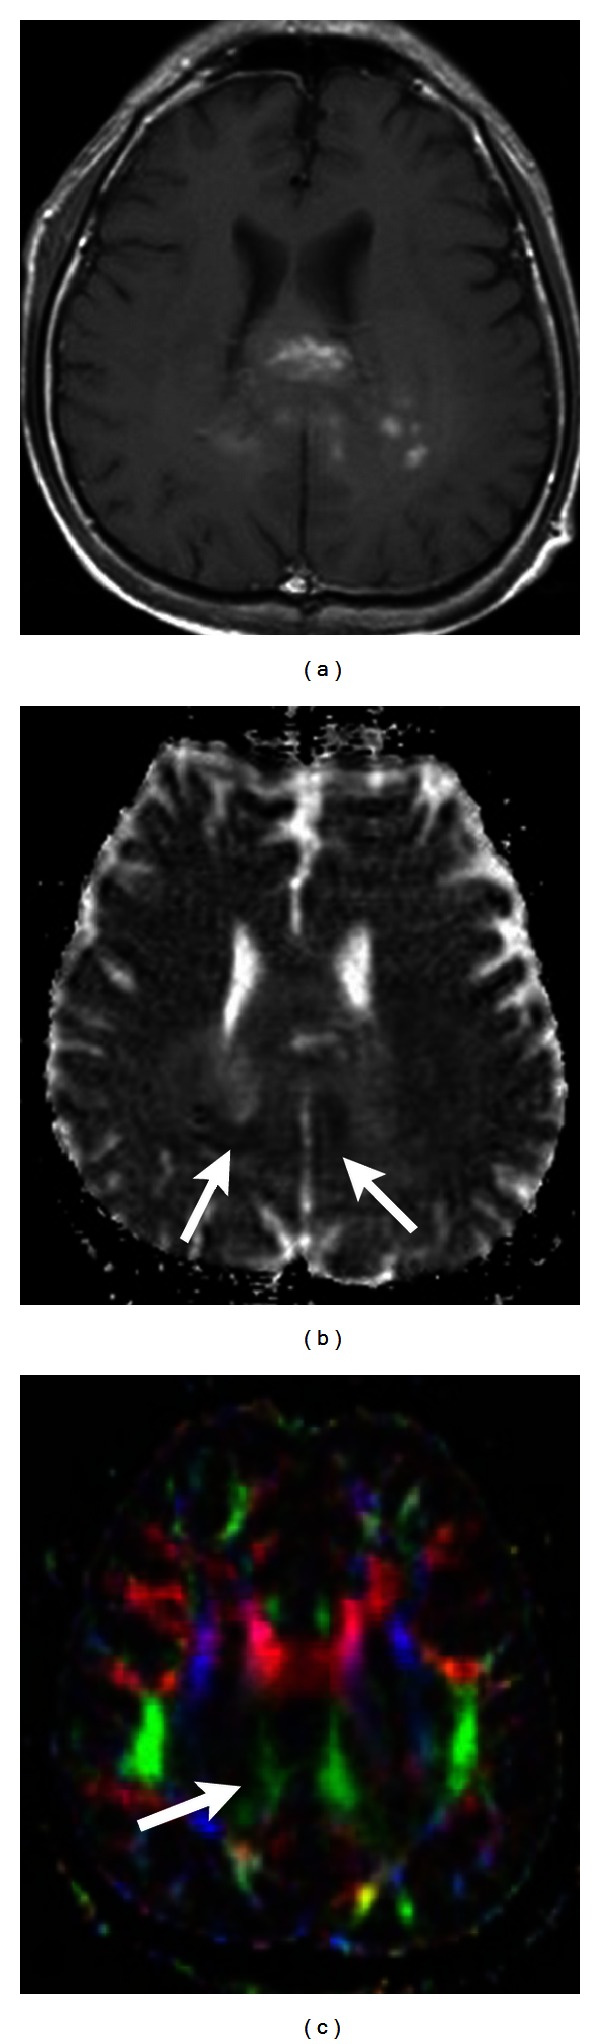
(a) Contrast-enhanced T1-weighted image demonstrates a butterfly glioblastoma involving the genu of corpus callosum with small areas of low ADC value on ADC map (arrows in (b)). On color-coded diffusion tensor imaging, the normal left-right-oriented red color (arrow in (c)) is lost due to destruction of the transverse tracts.
